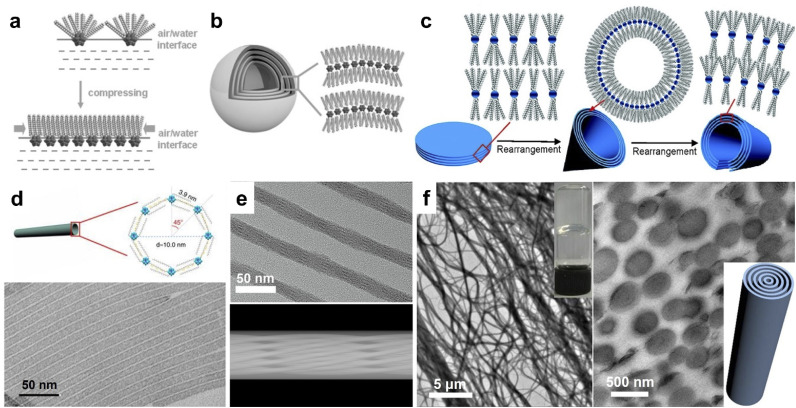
Amphiphilic POM assemblies. (a) Rearrangement of surface ligands on POM clusters at the air–water interface. (b) Onionlike spherical assembly of (DODA)_4_SiW_12_O_40_. Reproduced from ref. ^50^. (c) Reversible self-assembly of (DODA)_3_PW_12_O_40_/(DODA)_3_PMo_12_O_40_ between nanodisks, nanocones, and nanotubes. Reproduced from ref. ^52^. (d) [PW_12_O_40_]^3−^ single-walled nanotubes. Reproduced from ref. ^54^. (e) HRTEM image and 3D model of (TTA)_6_P_2_W_18_O_62_ helical nanotubes. Reproduced from ref. ^55^. (f) TEM image and cross section of (CTA)_3_(TBA)_3_P_2_W_18_O_62_ supramolecular gel. Reproduced from ref. ^56^.
